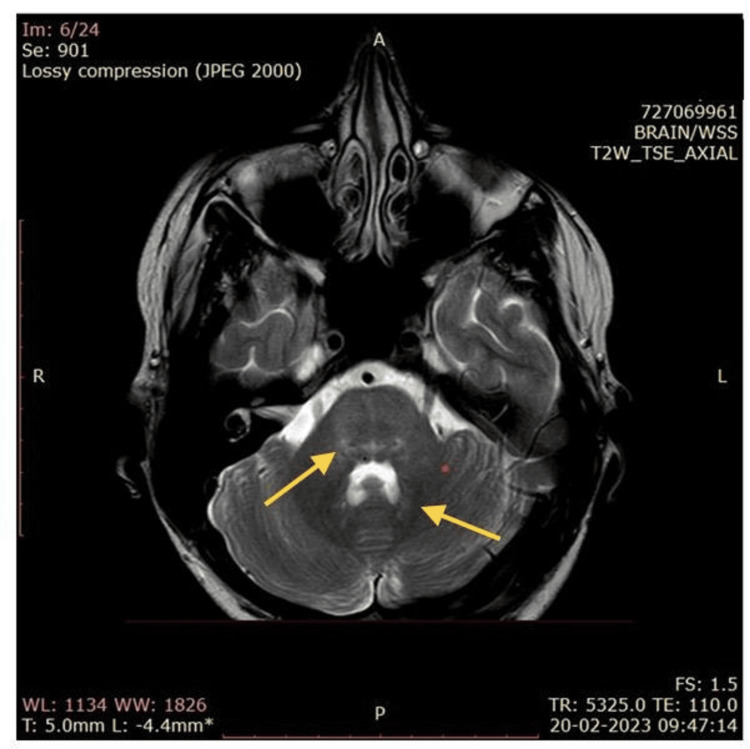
MRI of the head MRI showing minimal T2/flair hyperintense signal seen adjacent to the fourth ventricle at the dentate nucleus region of both cerebellum.
